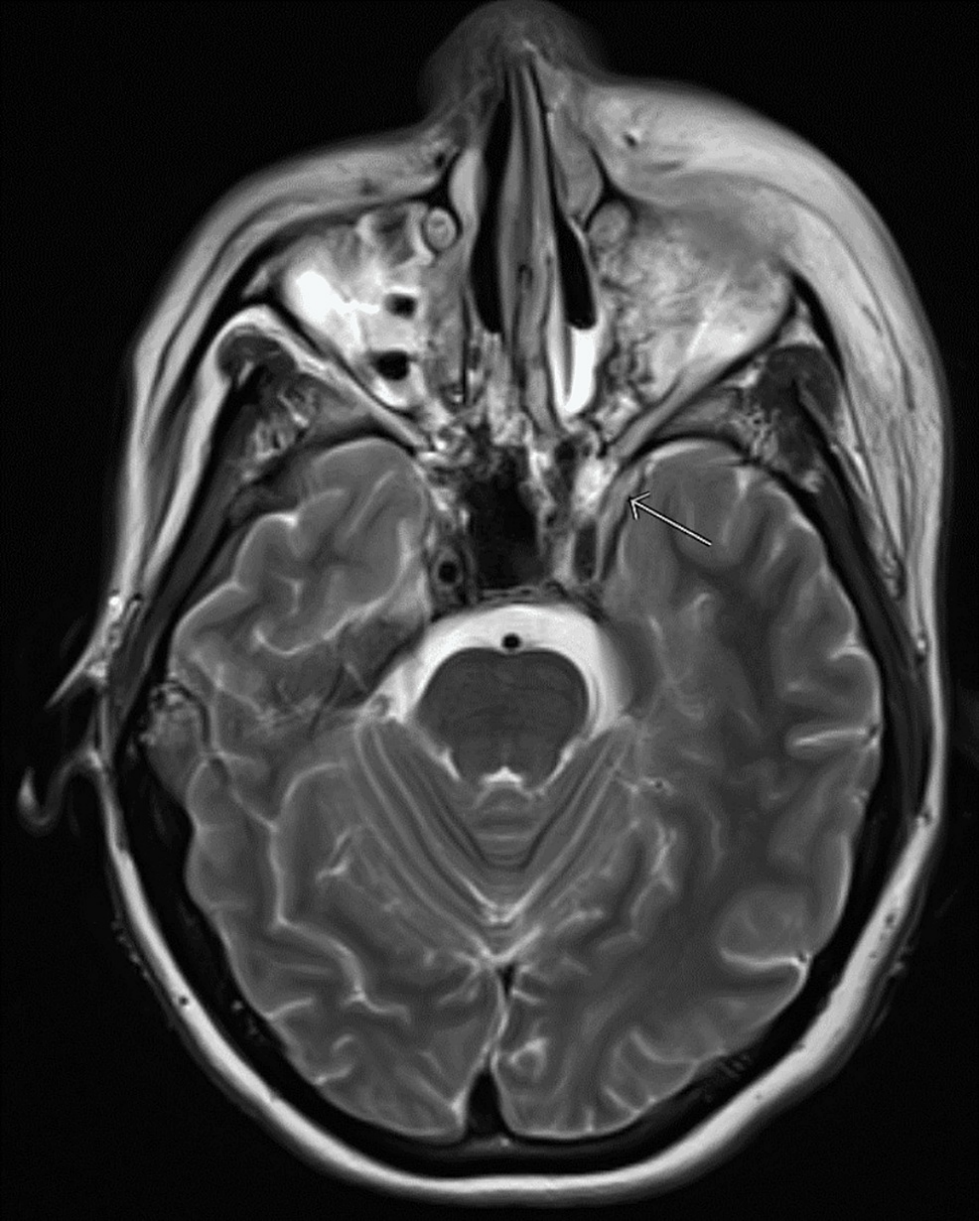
MRI depicting intracranial findings
